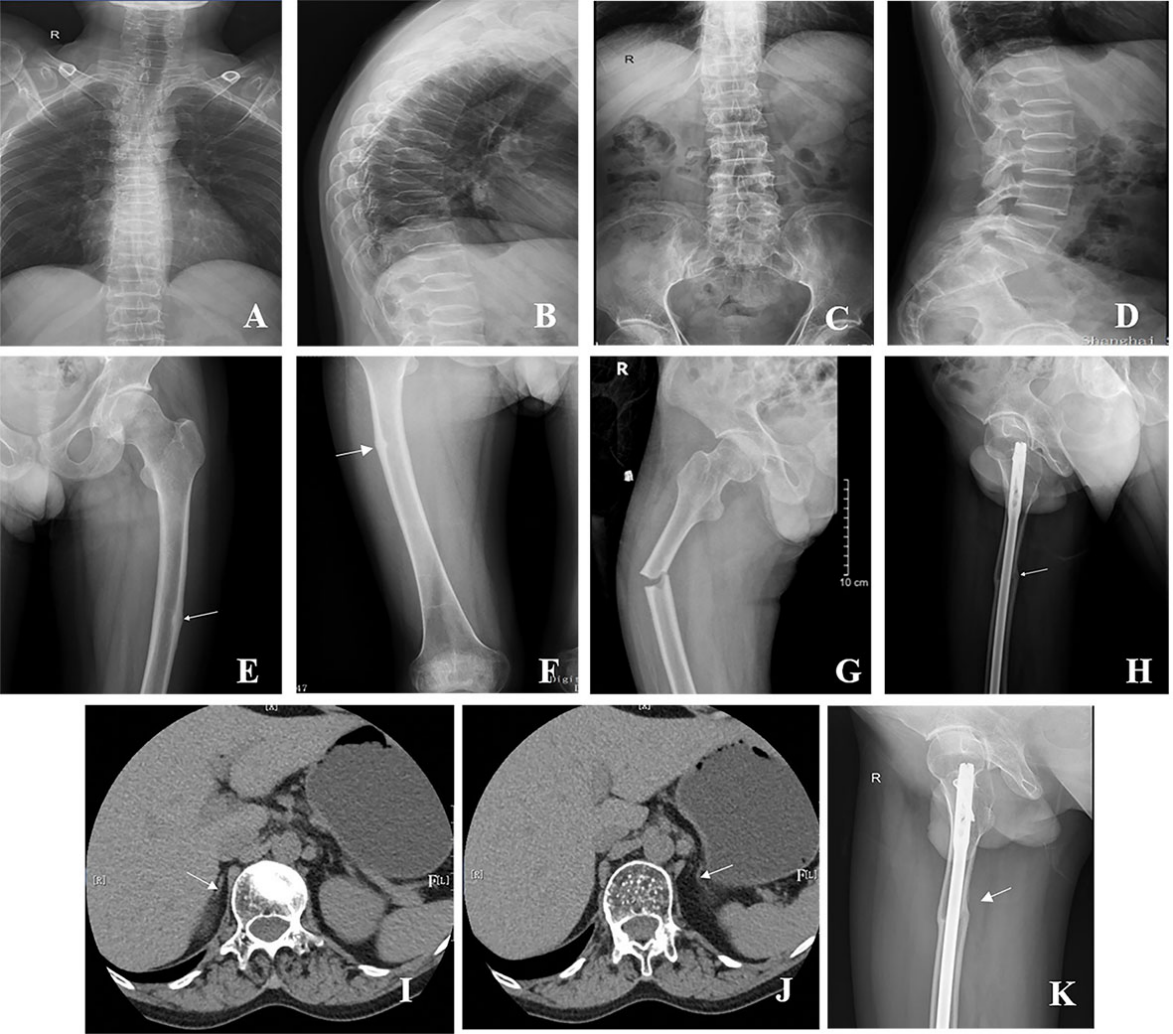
Radiographs of the patient with AHC. (A–D) Multiple vertebral compression fractures. (E, F) The white arrow shows the pseudofracture line in the left and right femurs. (G) Right femoral shaft fracture at the site of the pseudofracture line. (H) Right femoral shaft fracture after internal fixation. (I, J) The white arrow shows that the adrenal glands on both sides were small, and the structure was unclear. (K) Right femoral shaft fracture healing in 2022.
